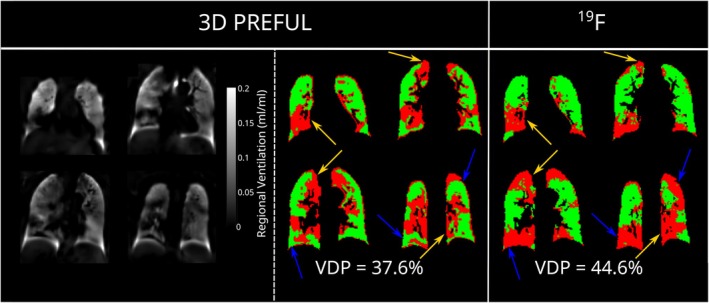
3D PREFUL MRI regional ventilation maps (on the left), respective ventilation defect maps (in the middle), and ^19^F (on the right) ventilation defect maps for a 60‐year‐old male with chronic obstructive pulmonary disease (FEV_1_ 53% of predicted value). Defect regions are color‐coded in red, gold arrows show the regional ventilation defect agreement, and blue arrows point out the regional differences. Sørensen–Dice coefficient was 61.8% and 48.2% for healthy and defect areas, respectively. FEV_1_, forced expiratory volume in 1 s; PREFUL, phase‐resolved functional lung.
